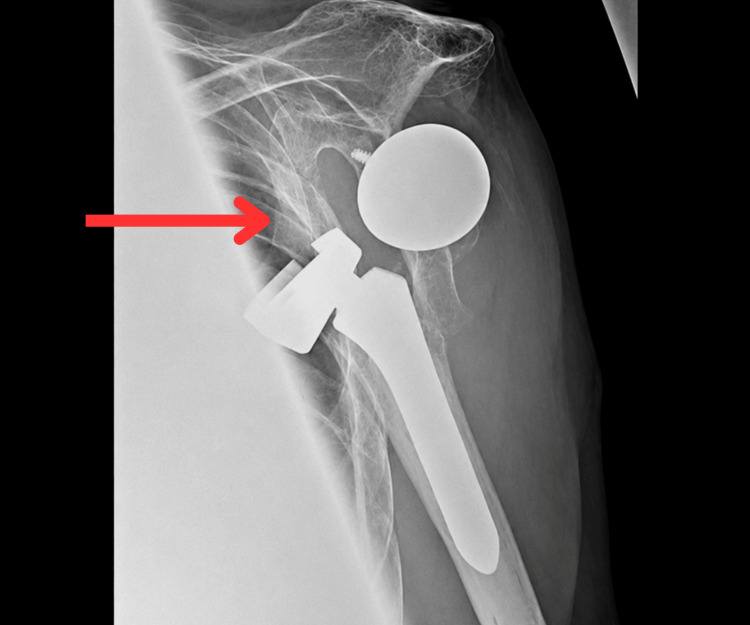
Radiograph confirming recurrent anterior dislocation prior to the second open revision
